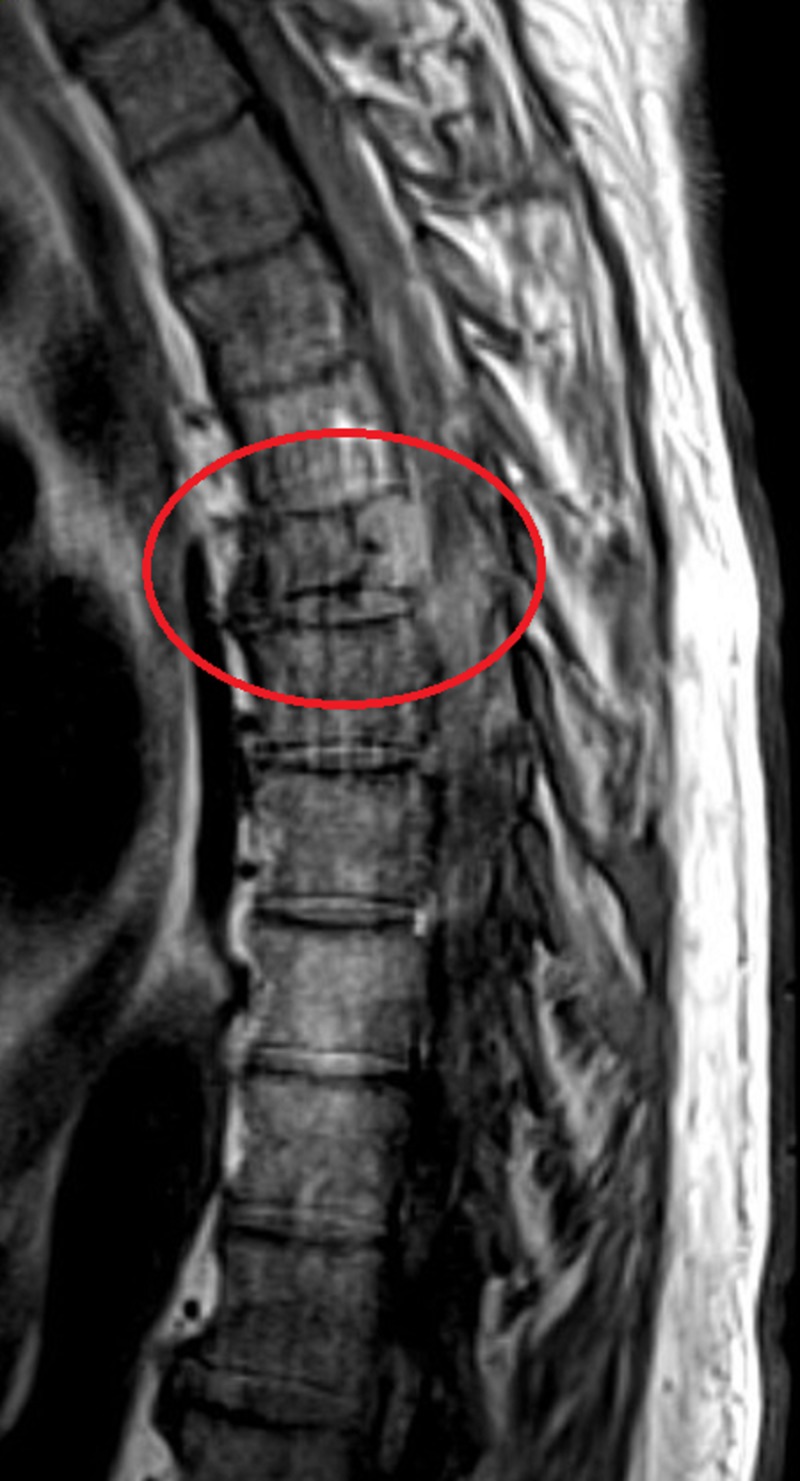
Magnetic resonance imaging of the thoracic spine with contrast A magnetic resonance imaging of the thoracic spine with contrast showing destructive osseous lesions in T6 and the transverse process on the left of T7 vertebral body
